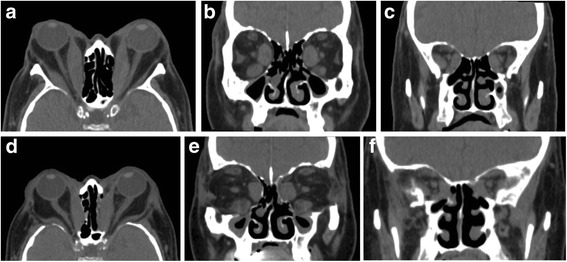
Preoperative and postoperative computed tomography (CT) of the orbit. Preoperative axial (a) and coronal (b and c) CT images showing proptosis, enlargement of extraocular muscles and apical crowding. Postoperative axial (d) and coronal (e and f) CT images showing the reduction in proptosis and relief of apical crowding
